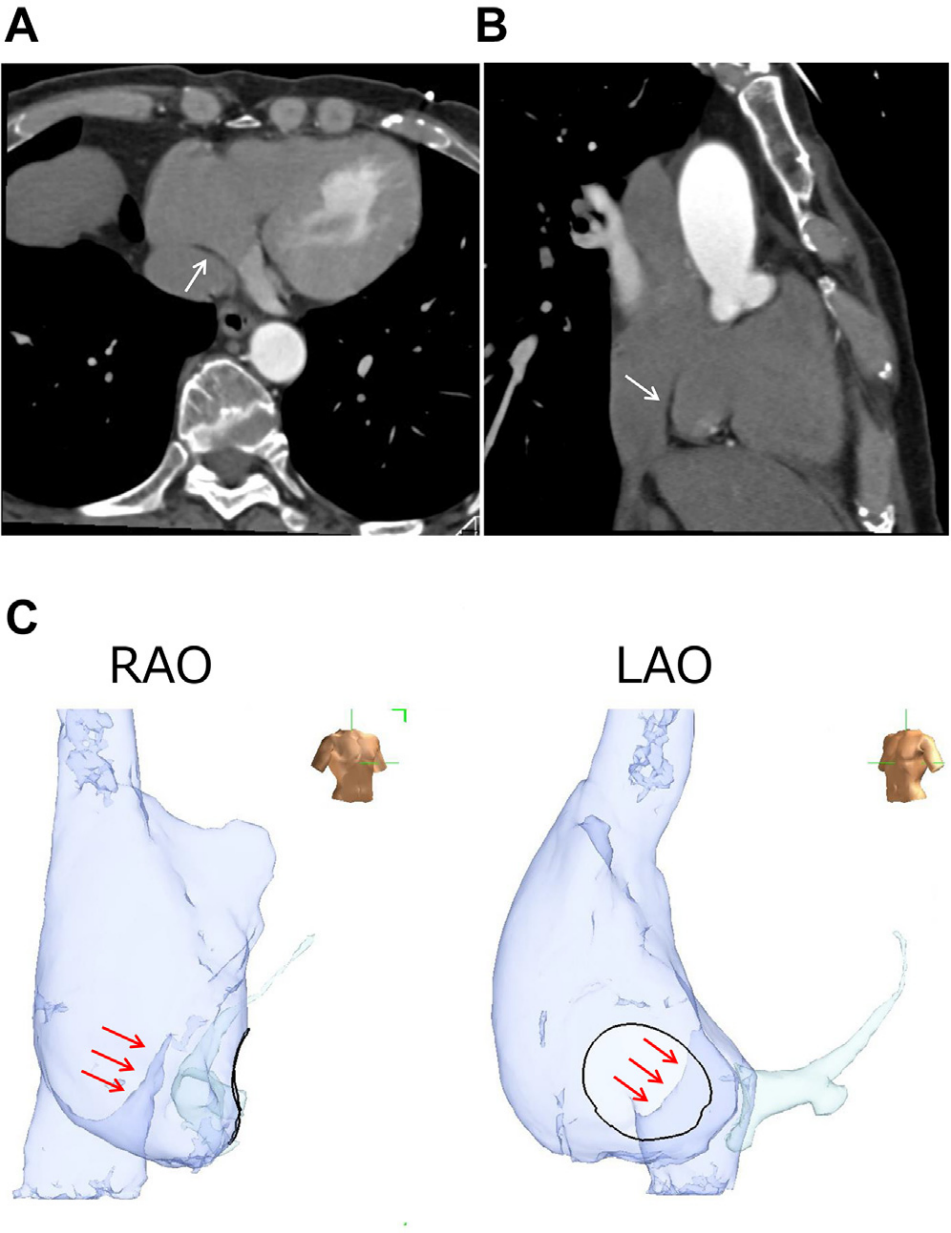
Computed tomography (CT) images. A: Axial view. B: Sagittal view. C: Three-dimensional CT image of cavotricuspid isthmus level. The prominent Eustachian ridge (*white and red arrows*) is noted. LAO = left anterior oblique; RAO = right anterior oblique.
